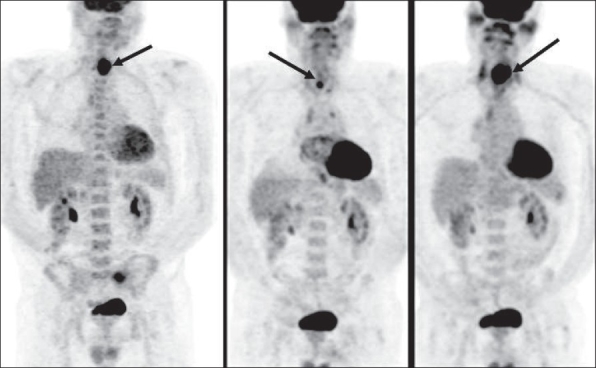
MIP images from three different PET studies of a 65-yearold man with laryngeal cancer, detected incidentally on a PET study (arrow in the left image) for a right lung mass (which was not FDG avid and was proven to be benign). A repeat PET study (middle image) 3 months after completing concurrent chemoradiation therapy shows significant but incomplete response with some residual disease (arrow). However, biopsy failed to reveal residual disease and the patient was followed routinely without additional therapy. Five months later, the patient required emergent admission for a tracheostomy for shortness of breath. Vocal cord biopsy revealed malignant disease and a subsequent PET study (right image) shows significant progression of disease (arrow).
